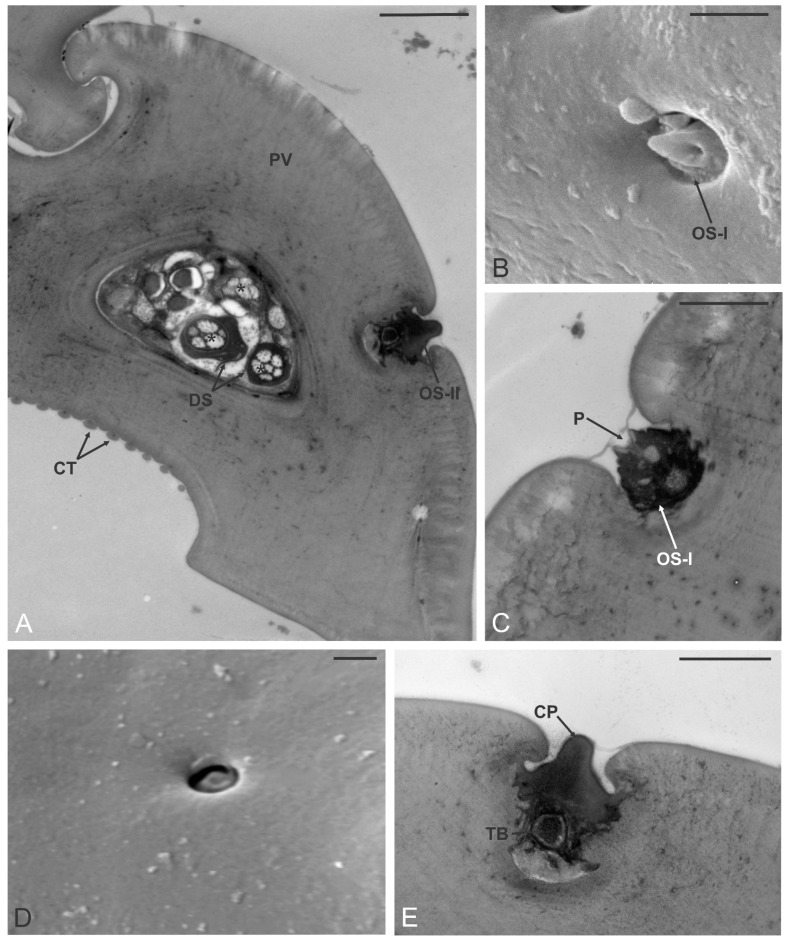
Dryocosmus kuriphilus* ovipositor. (A) TEM cross-section of one of the paired valves (PV): the external side presents a single ovipositor sensillum type II (OS-II), while the internal side is occupied by a single row of ctenidia (CT). The PV lumen reveals the presence of three bundles of sensory neurons (*), each one comprised of 5–6 sensory neurons enclosed in a thick dendrite sheath (DS). (B) SEM close-up view of an OS-I: a well evident apical pore is observed. (C) TEM longitudinal section of OS-I: the apical pore (P) is revealed. (D) SEM picture of an OS-II. (E) TEM longitudinal section of an OS-II: the peg (CP) is made of solid cuticle without pores and reveals the presence of a single sensory neuron ending in a tubular body (TB). Bar scale: (A), 2 µm; (B–E), 1 µm.
